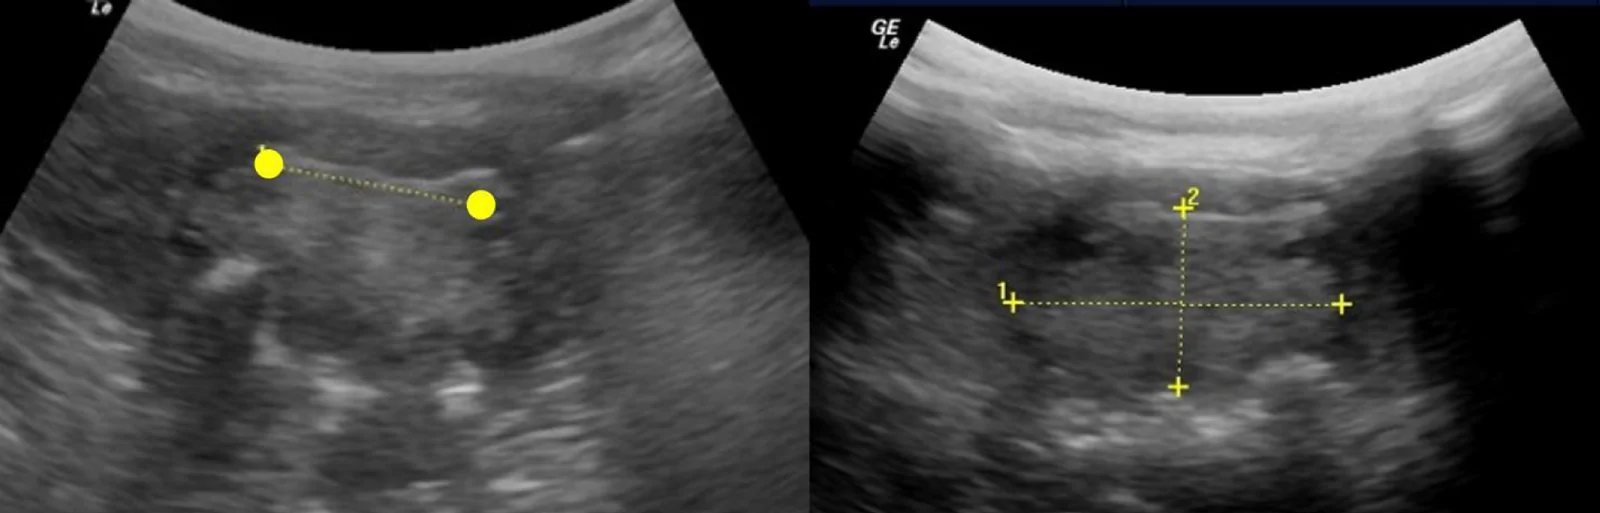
Oropharyngeal ultrasound in children. Measurement of the distance between the lingual arteries at the base of the tongue (left) and measurement of the width (1) and height (2) of the tongue at its base at the back of the oropharynx (right).
